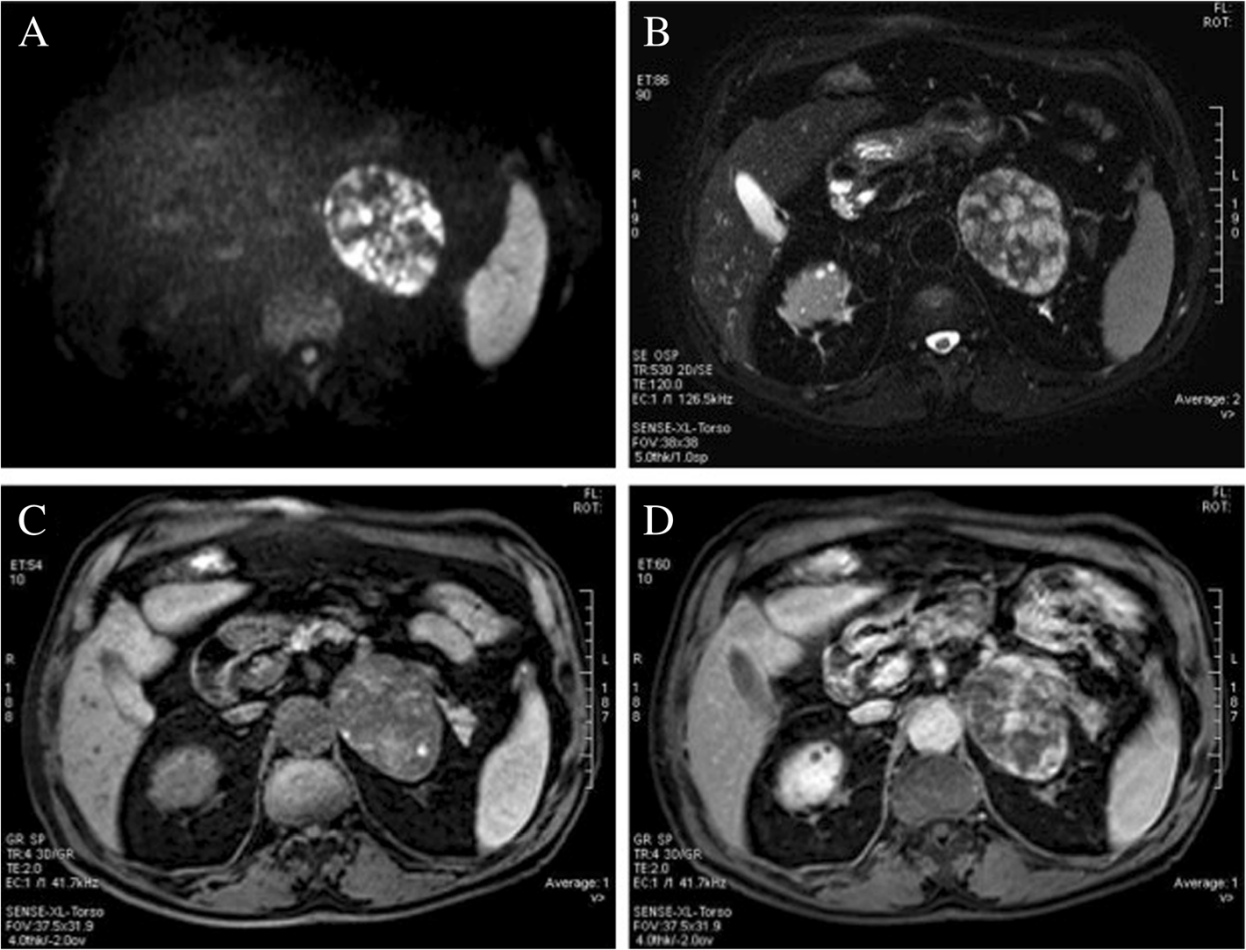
MRI shows restriction of intralesional molecular water diffusion in Diffusion Weighted Imaging (a), high signal intensity intralesional areas in T2 fat-saturated weighted image (b), high signal intensity intralesional areas in T1 fat-saturated weighted image (c) with inhomogeneous enhancement in contrast-enhanced T1 fat-saturated weighted image (d)
